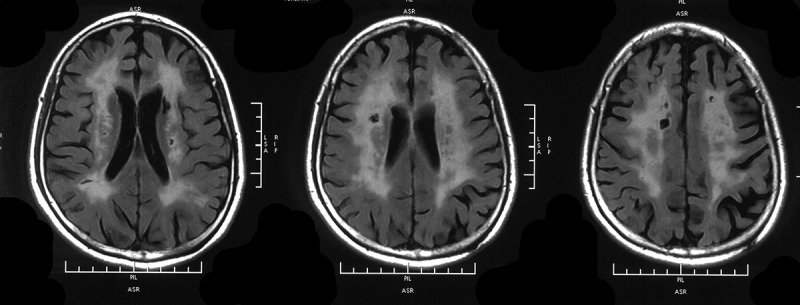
Head MRI and FLAIR images of III6. Image shows bilateral lateral ventricles, bilateral semi-oval center with multiple abnormal signals, and demyelination in the white matter around the bilateral lateral ventricles.
